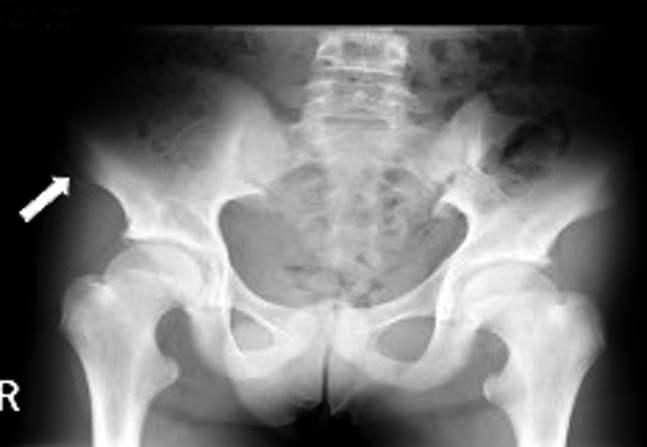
X-ray of the pelvis. A slightly displaced radiopaque structure was detected on the lateral side of the ASIS, suggesting an ASIS avulsion fracture (arrow).
